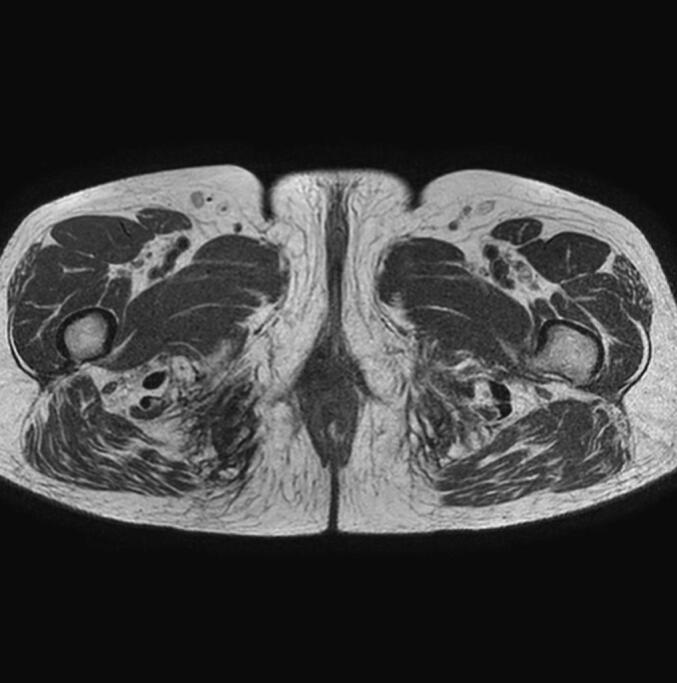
Complete surgical healing was achieved after the last MRI control.
